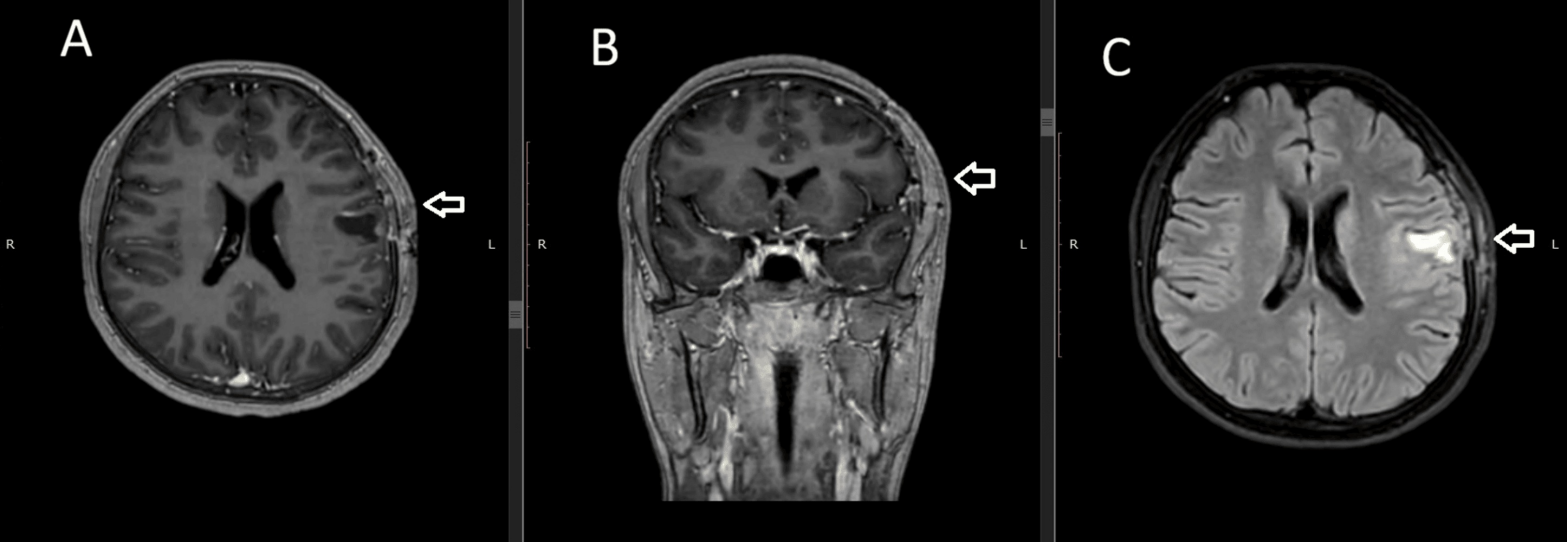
Postoperative brain MRI at two-month follow-up. (A) Axial T1-weighted image and (B) coronal T1-weighted image demonstrating postoperative changes in the right frontotemporal region with no evidence of residual or recurrent tumor (arrows). The surgical craniectomy defect is visualized. (C) Axial T2-FLAIR image showing expected postoperative gliosis and encephalomalacia at the surgical site (arrow). FLAIR, fluid-attenuated inversion recovery
